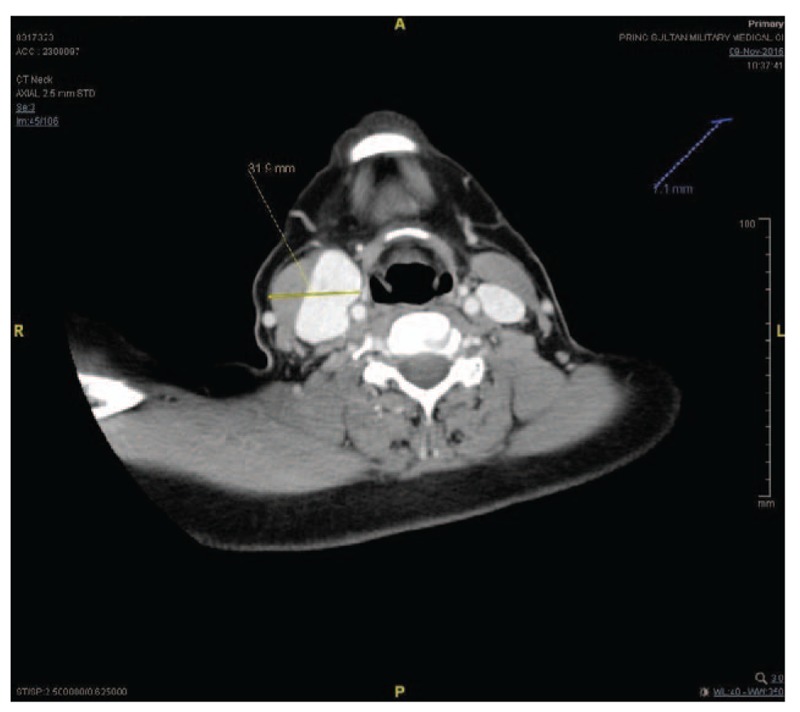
Computed tomography scan (axial view) showing an anterolateral filling defect, which could represent a thrombus.
